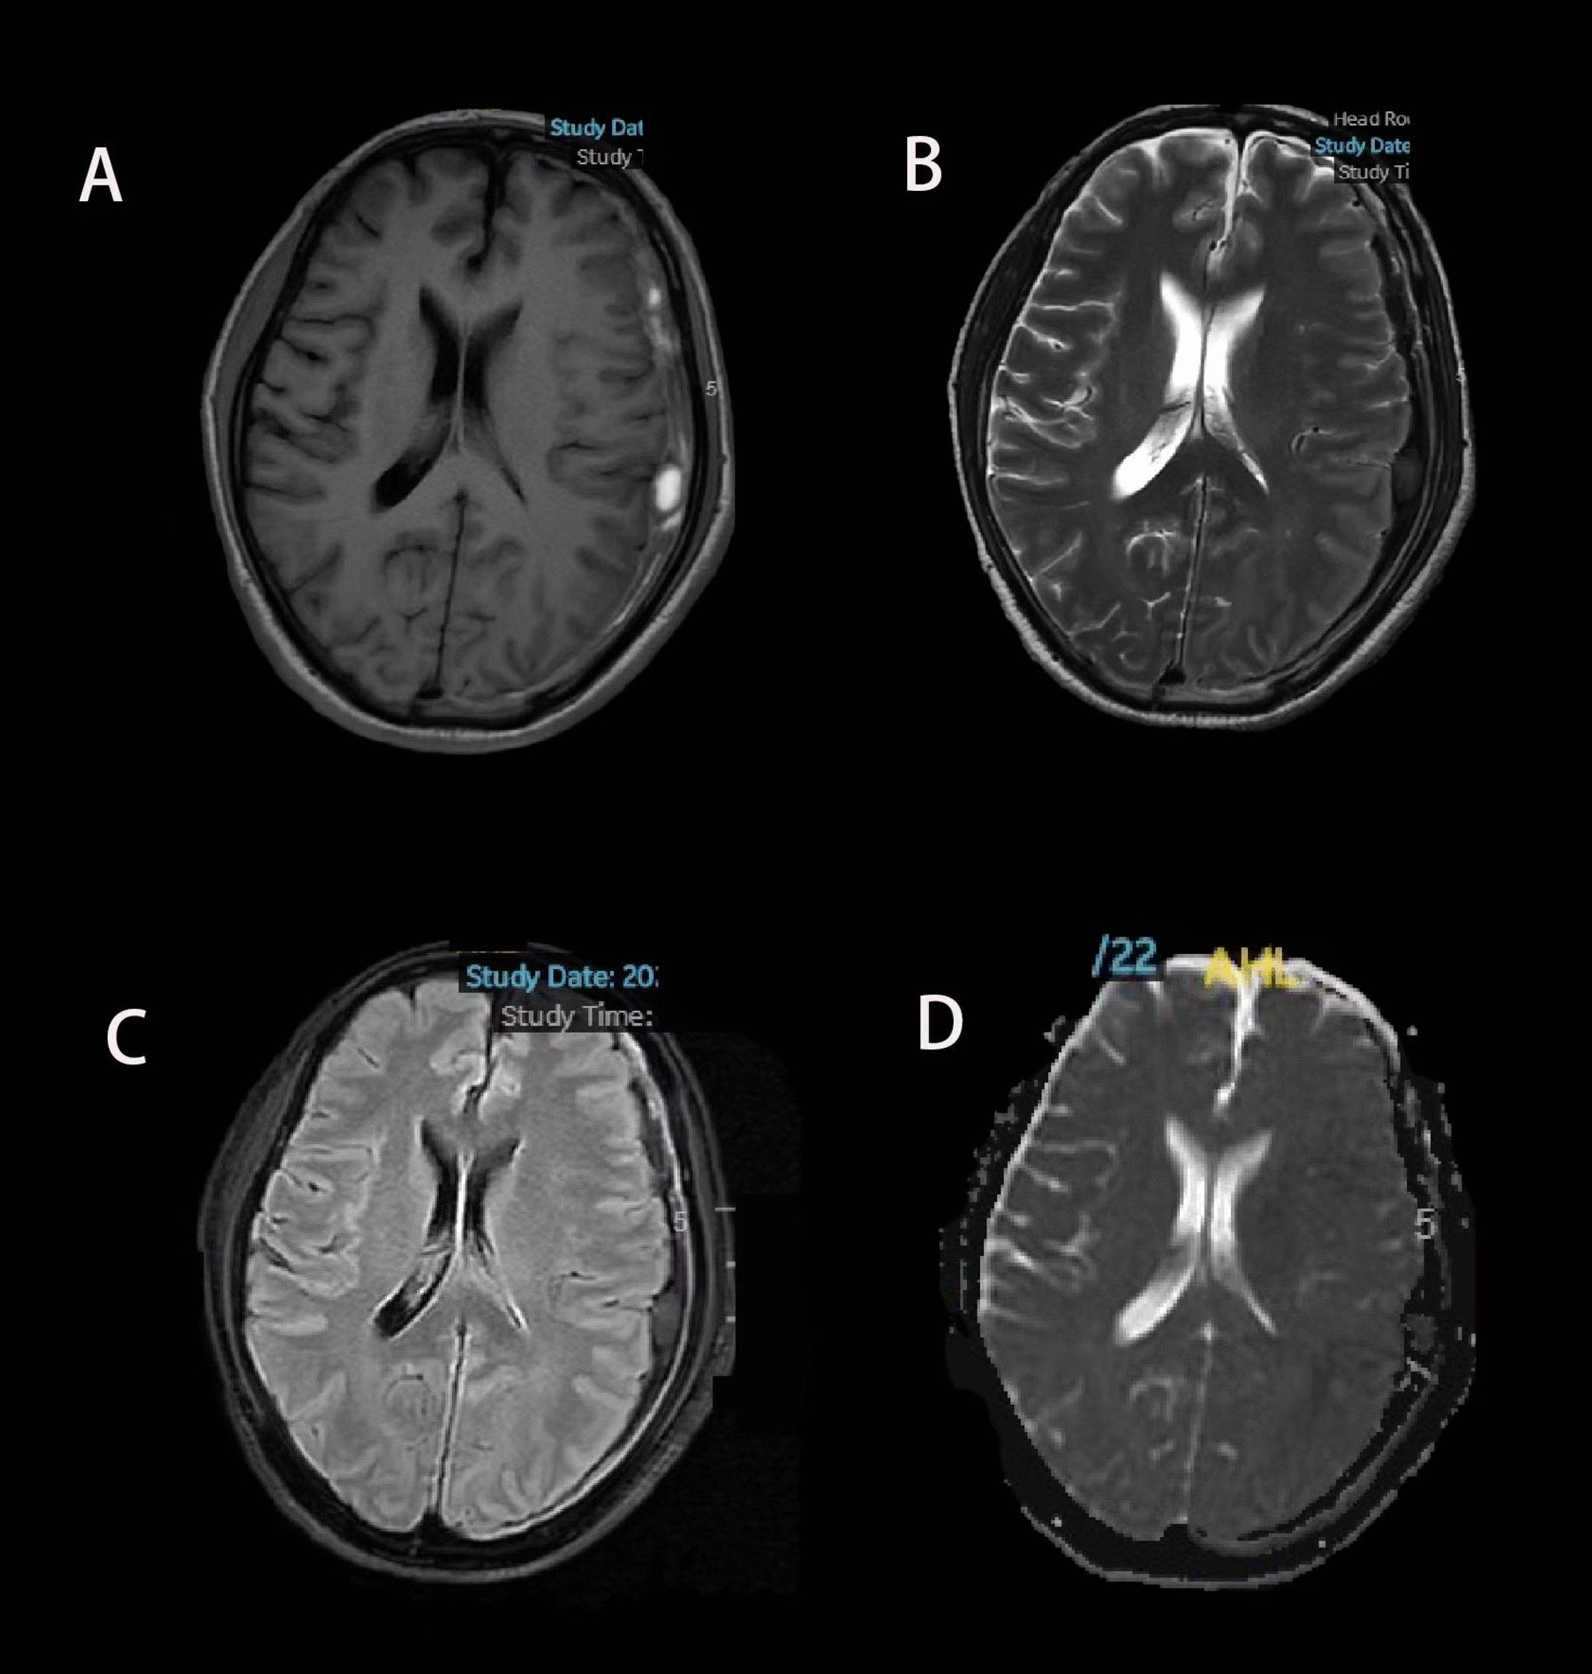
Magnetic resonance imaging of the brain on admission. Axial T1-weighted (A), T2-weighted (B), T2-weighted fluid attenuated inversion recovery (C), and apparent diffusion coefficient (D) sequences of the brain magnetic resonance imaging (MRI) on admission revealed left subdural hemorrhage
